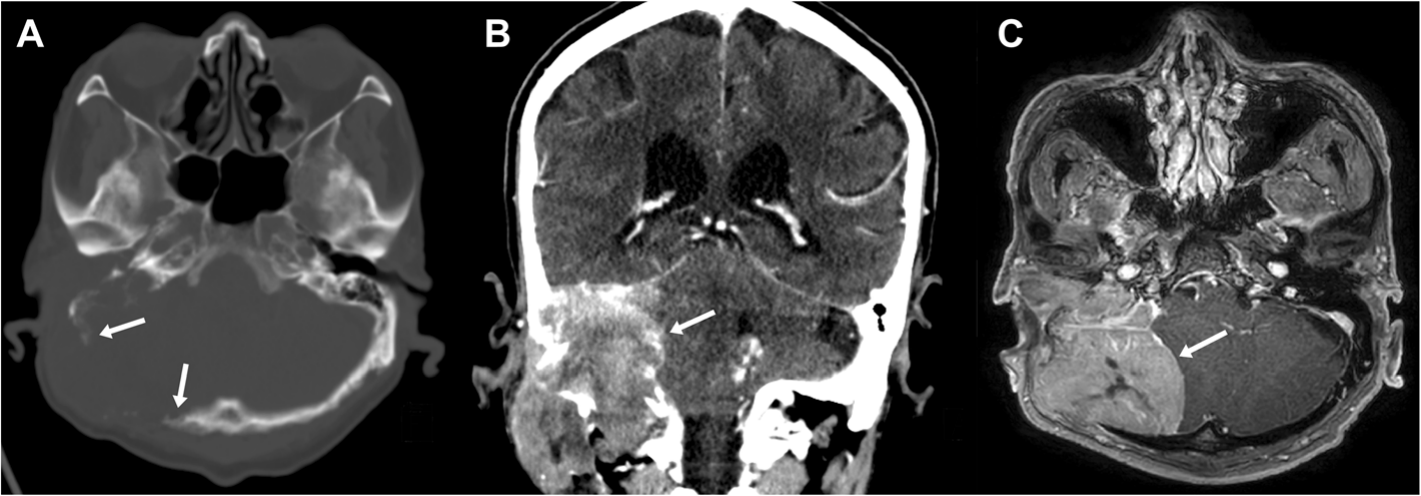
Preoperative imaging. Axial computed tomography (CT) scan with bone window (a) shows a destructive osteolytic mass lesion in the right temporal-posterior fossa region. Coronal CT scan (b) and magnetic resonance imaging (c) revealed an 8 × 7 cm homogenous mass extending to the cervical region
